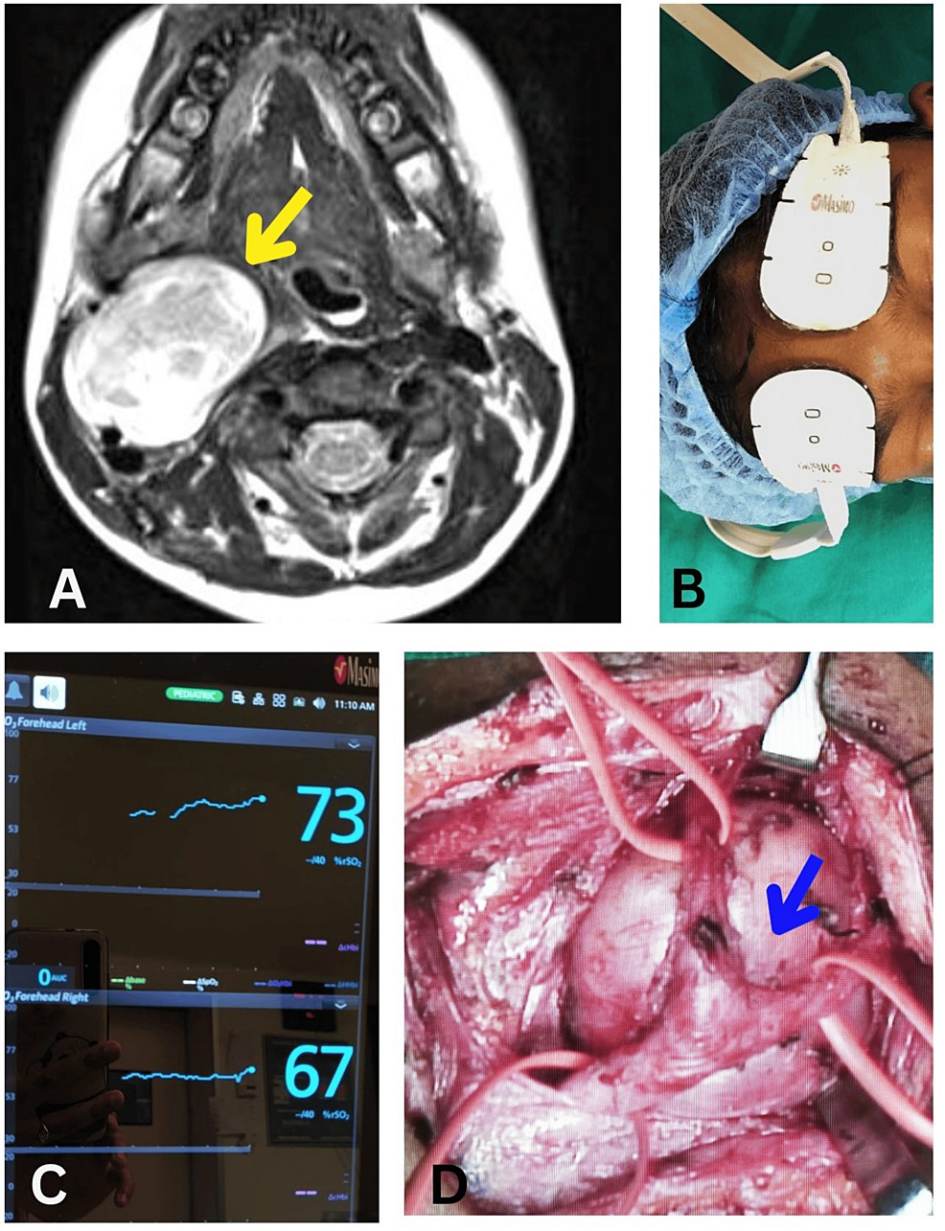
A: MRI displaying the mass in the carotid space (*yellow arrow), splaying the right internal and external carotid arteries). B: NIRS monitoring electrodes attached to the patient. C: Baseline recording of NIRS. D: Surgical location of the tumor (posterior to the bifurcation of the common carotid artery). *blue arrow
